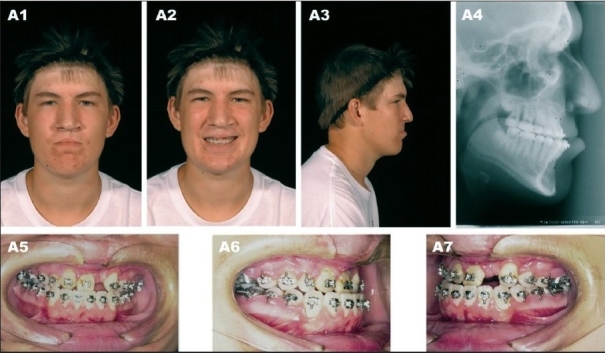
(A) A 17 year-old patient with bilateral complete cleft lip/nose, alveolus and palate after completion of growth and before orthognathic surgery. (A1) Frontal view (A2) Frontal smiling (A3) Right lateral view (A4) Preoperative lateral cephalometric roentgenogram (A5) Frontal view with orthodontic brackets (A6) Right oblique view with orthodontic brackets (A7) Left oblique view with orthodontic brackets
